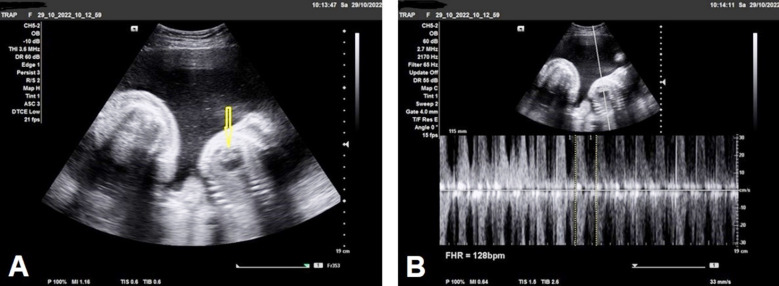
(A) Gray-scale ultrasound study shows two intrauterine fetuses in a single amniotic cavity, the right twin appears structurally normal-developed with no gross congenital (pump fetus), (arrow). The left twin appears structurally abnormal with no visible cardiac tissue or activity (acardiac fetus). (B) Doppler ultrasound shows two intrauterine fetuses in a single amniotic cavity, the right fetus shows positive regular cardiac activity.
